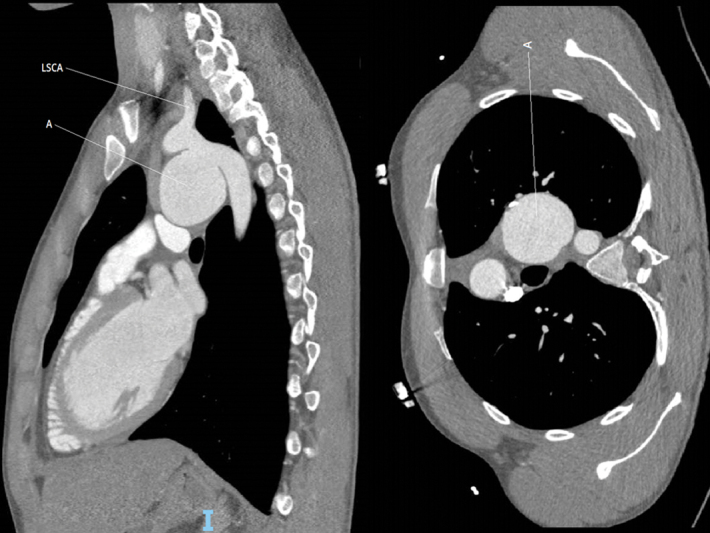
Computed tomography scan of patient 2. A, aneurysm; LSCA, left subclavicular artery.
